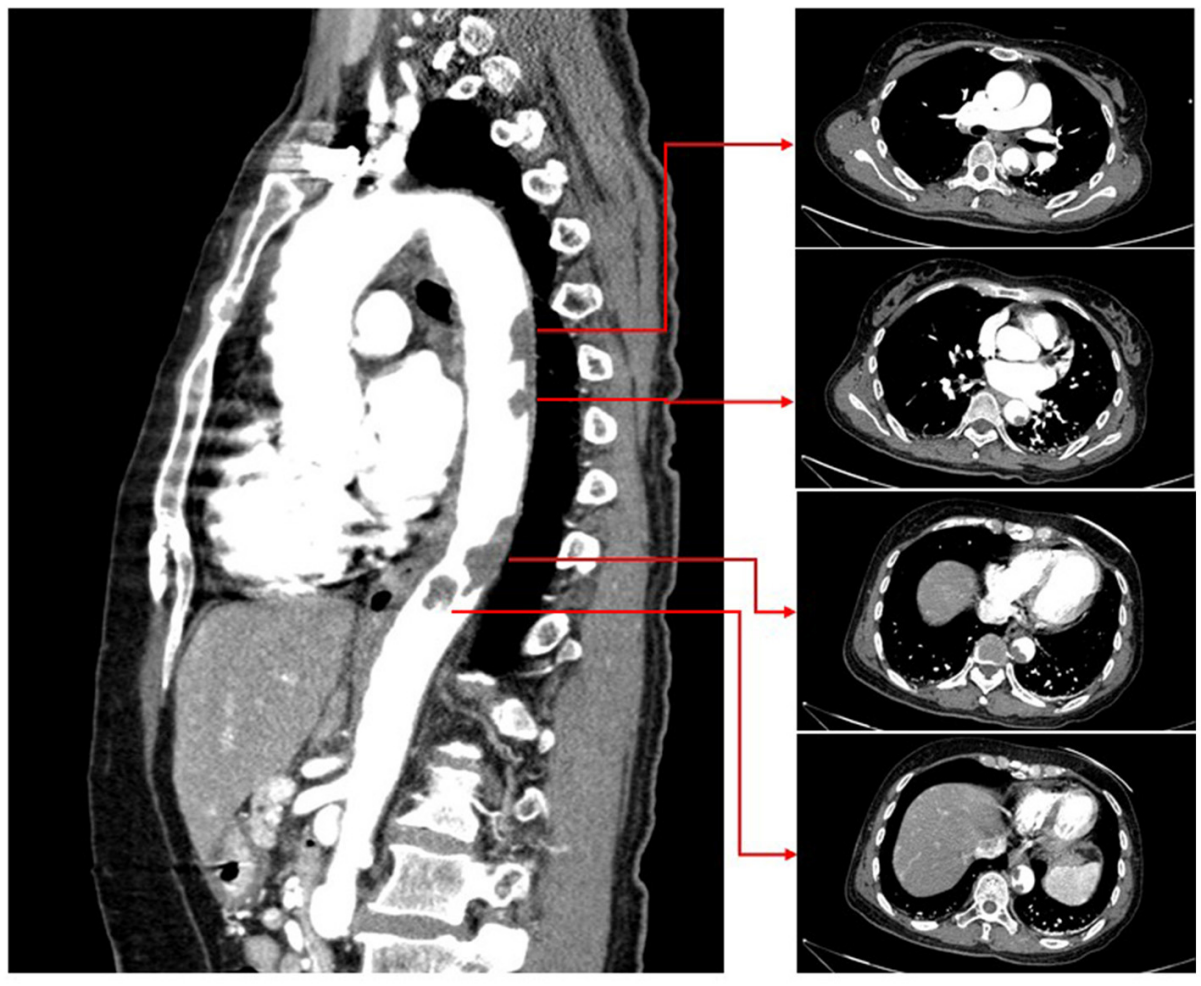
CT angiography revealing massive mural thrombus in the descending aorta.
